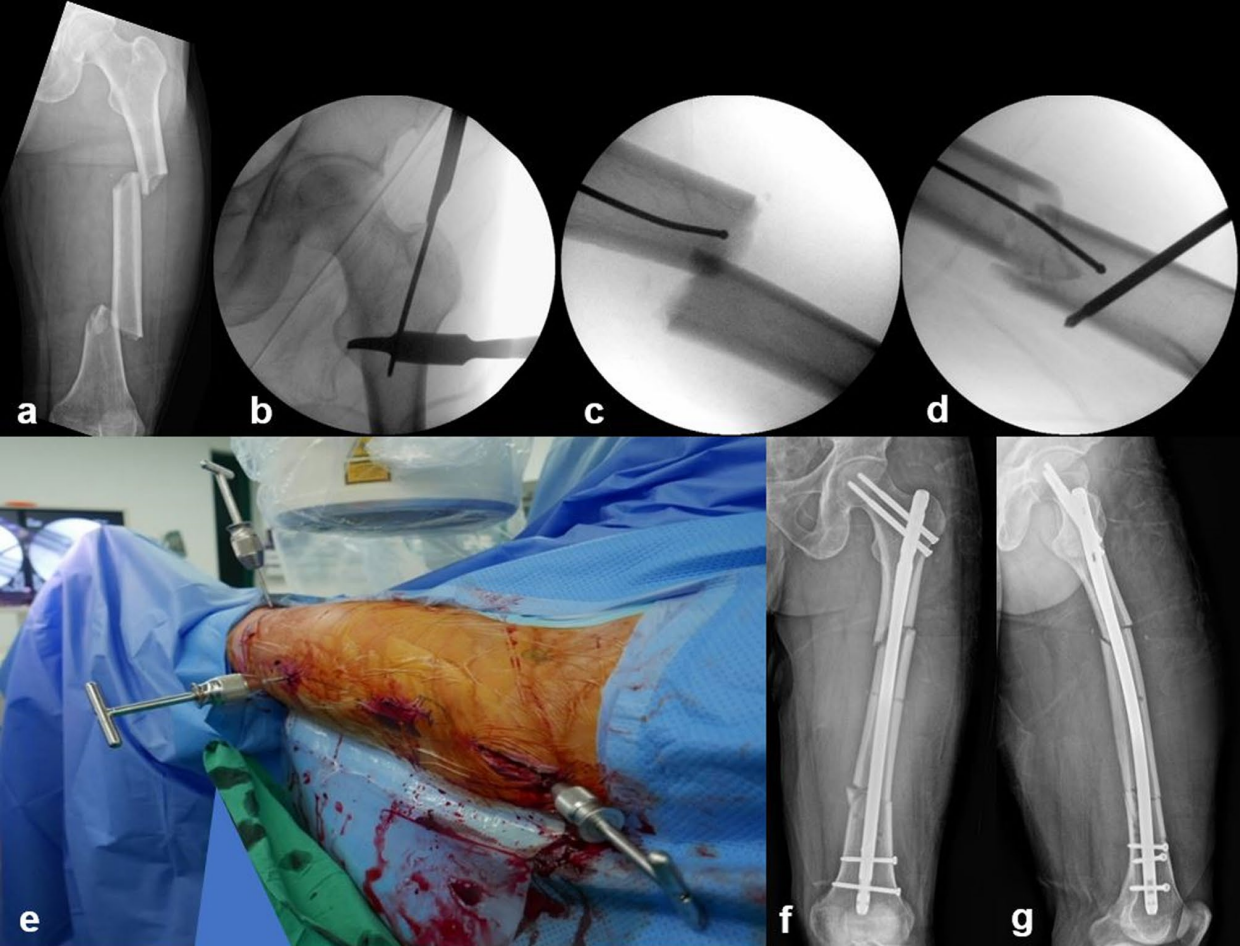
A 43-year-old male patient with a left femur segmental fracture caused by a motorcycle accident. (a) Plain radiograph at the initial visit. Segmental fracture was observed around the isthmus of the femur. (b) To aid reduction, a minimal skin incision was made in the lesser trochanter area, and the Hohmann retractor was placed on the lesser trochanter and pressed down to negate the flexion deforming force. (c) It was difficult to achieve reduction by a closed mean due to the absence of bony contact at the fracture site. (d) A percutaneous incision was made and a Schanz pin was inserted to manipulate the fracture site using the joy stick technique. (e) Intraoperative clinical photograph of the joystick technique. (f, g) Plain radiographs after surgery show good quality of reduction, restoring alignment, and the length of femur.
